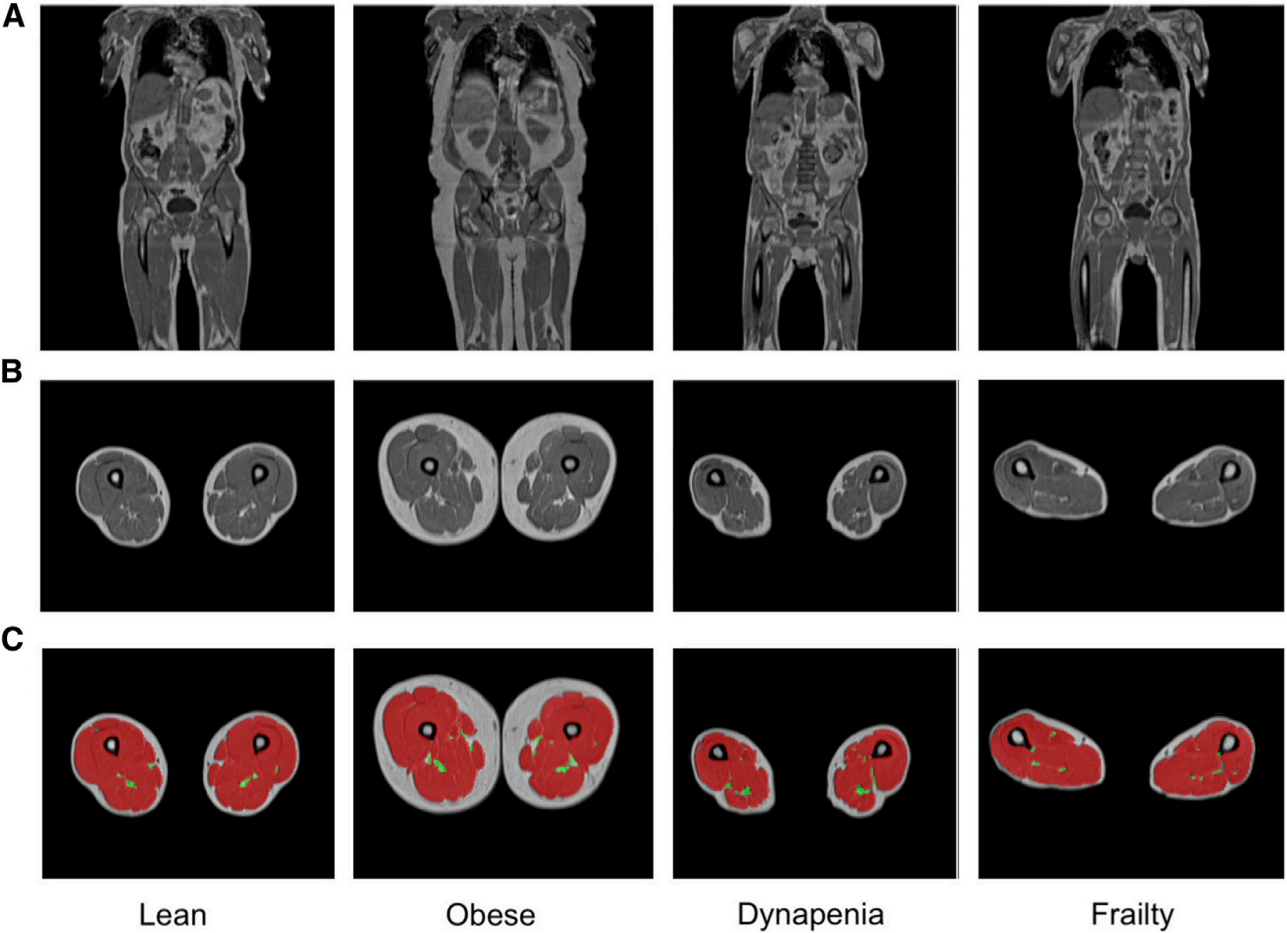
Representative samples of (A) coronal and (B) axial MRI views and (C) the total muscle (red) and the thigh IMAT (green) segmentations overlaid on the axial views, obtained from lean, obese, dynapenic and frail participants from the Dixon acquisition.
